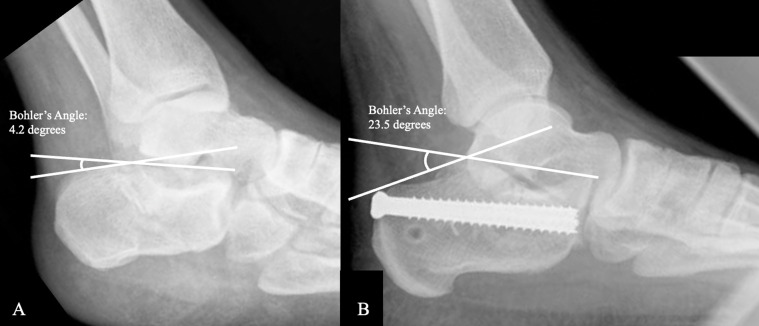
(A) Preoperative lateral foot radiograph of joint depression-type calcaneal fracture. Bohler’s angle measured 4.2 degrees through intersecting lines tangential to the superior tuber and the superior aspect of the posterior facet, and the superior aspect of the posterior facet and the anterior calcaneal process. In (B) a postoperative lateral foot radiograph is shown status post closed reduction with percutaneous fixation with two cannulated, fully threaded 6.5 mm screws with restoration of Bohler’s angle measuring 23.5 degrees.
